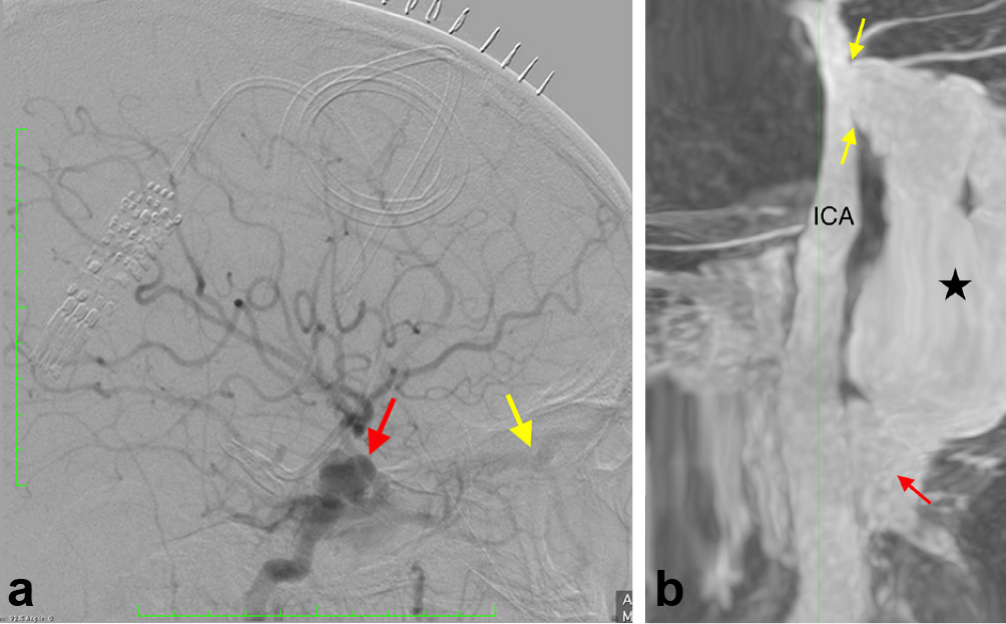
Aangiographic and post processed reconstructed CBCT image, showing (a) right
